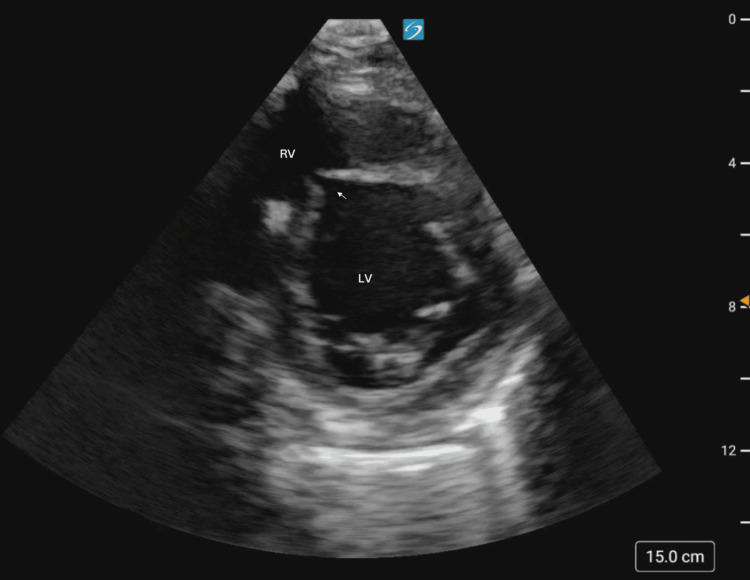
Parasternal short axis view, redemonstration of ventricular septal defect with arrow indicating VSR VSR: Ventricular septal rupture
